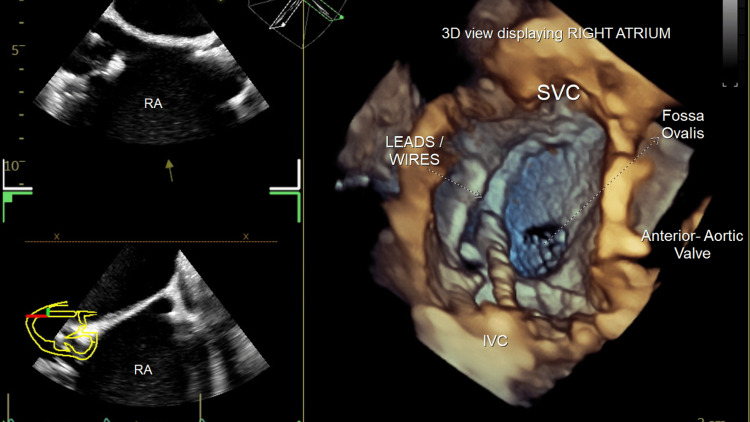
Three-Dimensional Transesophageal Echocardiographic Visualization of a Retained ICD Lead Within the Right Atrium Three-dimensional transesophageal echocardiography demonstrates the retained intracardiac lead traversing the right atrium with its relationship to adjacent structures, including the superior vena cava (SVC), inferior vena cava (IVC), and interatrial septum. Enhanced spatial visualization helped define lead orientation and intracardiac position before attempted snare retrieval. ICD: implantable cardioverter-defibrillator
